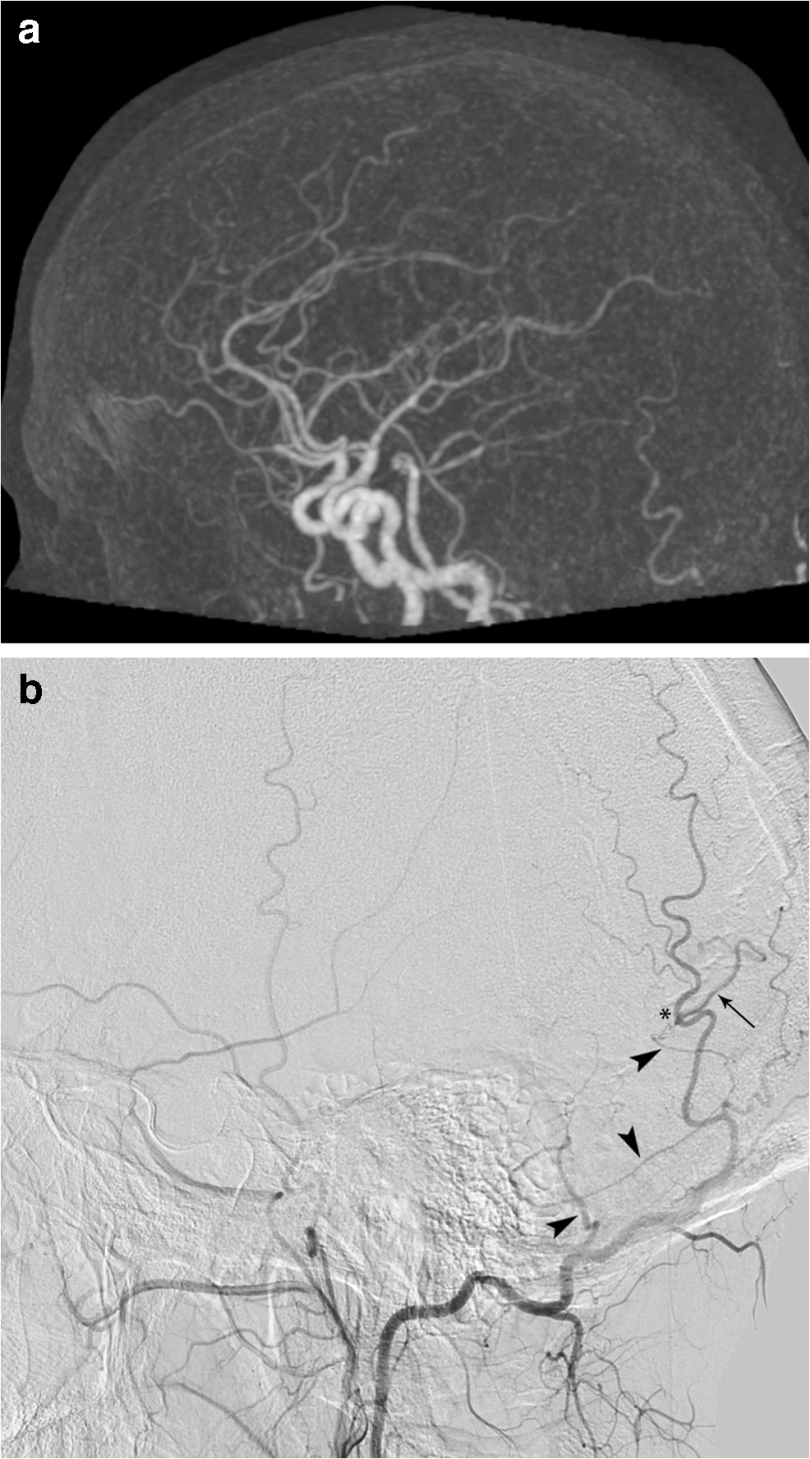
Panels a and b (right external carotid artery injection) are from the case where a false-negative 4D-CTA missed a dAVF. The DSA demonstrates a small transosseous dural branch from the occipital artery (arrowheads) feeding a Borden-type 3 dAVF (asterisk). The arrow depicts the arterialized draining vein. Some of these structures are partly seen in the 4D-CTA study, but their significance is unclear
